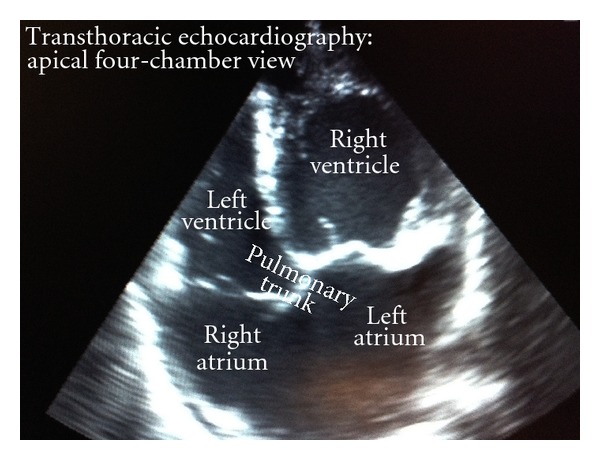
The transthoracical echocardiography (here apical four-chamber view) demonstrates the in situ anatomy in L-TGA. Both ventricles changed their position. The right ventricle shows dilation, hypertrophy, and trabecularisation. The right atrioventricular valve is located closer to the apex compared with the left atrioventricular valve.
